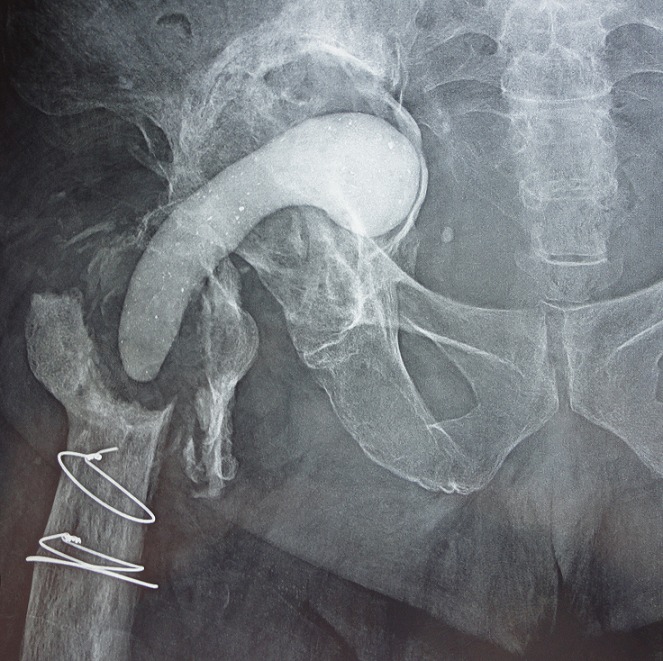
Radiographie stand d'un patient qui présente un sepsis tardif sur prothèse totale de la hanche avec ablation de prothèse et mise en place d'un spacer au ciment antibiotique
